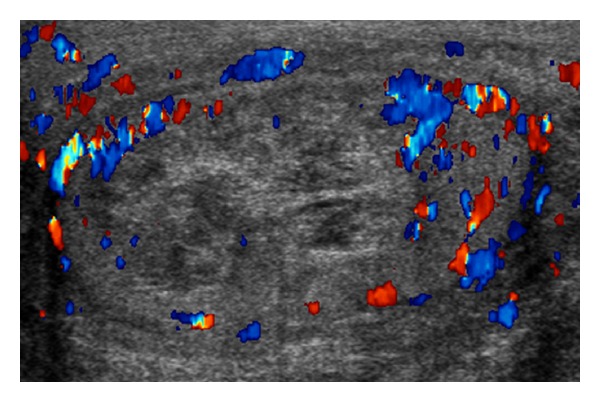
Postoperative scrotal ultrasound.
